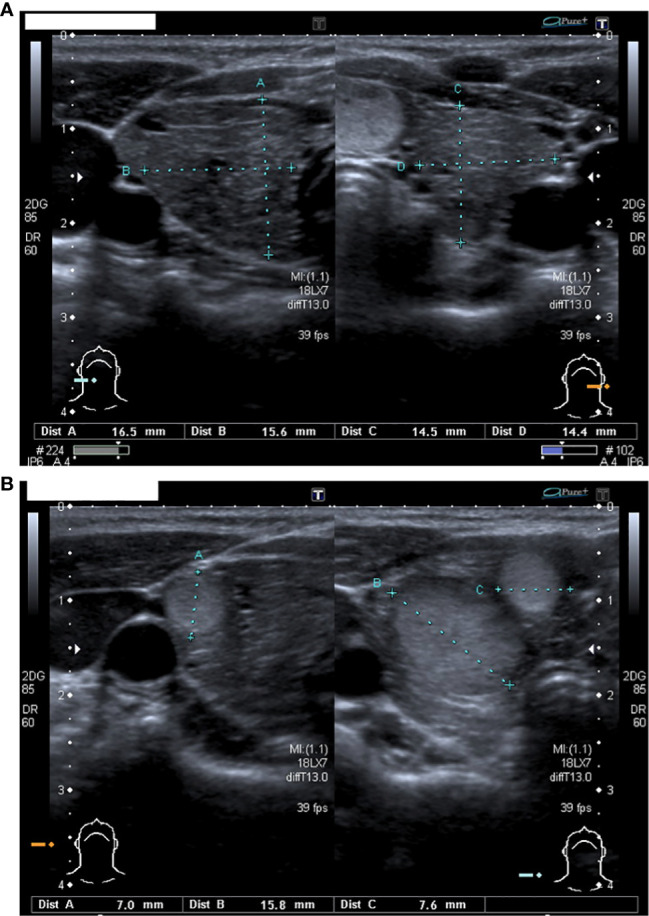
(A) The thyroid ultrasound performed at 6 years of age: an enlarged and non-homogeneous echotexture gland with increased antero-posterior and transversal diameters (16,5*15,6 mm for right lobe and 14,5*14,4 mm for left lobe). (B) Nodules in the right lobe.
